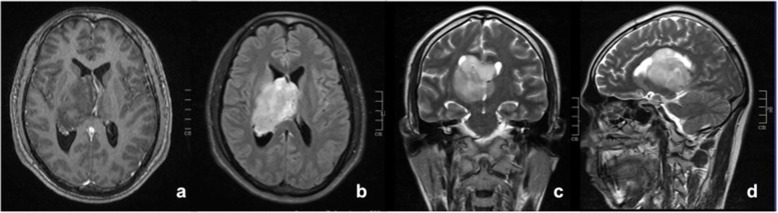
MRI brain (contrast) showed a large, fairly defined lobulated heterogeneously enhancing space occupying lesion with its epicenter in subependymal region of right lateral ventricular body, showing gross extensions into the intraventricular space, thalamus, body of corpus callosum, and leftward displacement of septum pellucidum, with mild ventricular dilatation. There was a small eccentric non-enhancing cystic necrotic focus. A small focus, hypointense on T1 and hyperintense on T2 and FLAIR sequences with restricted diffusion on diffusion-weighted imaging (DWI) and heterogeneous post-contrast enhancement was seen involving left thalamus. No leptomeningeal enhancement was seen. a Axial T1 contrast sequence. b–d Axial, coronal, and sagittal T2 FLAIR sequences of the brain showing the tumor extensions
